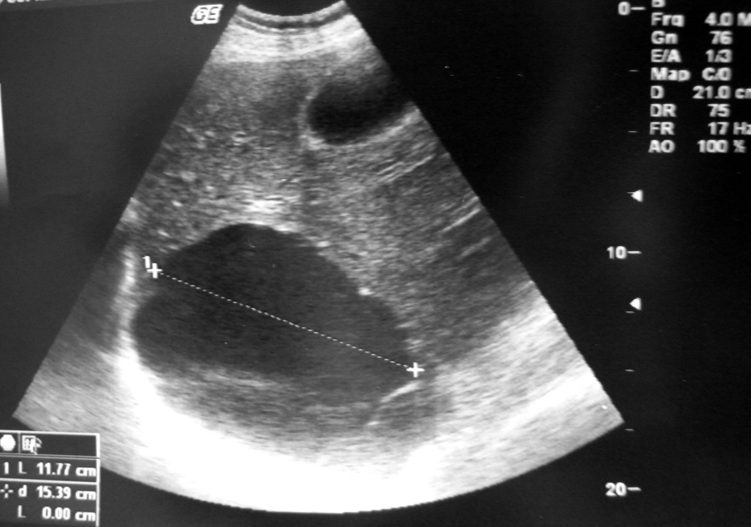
During first admission. Abdominal ultrasound revealing a sizeable right liver lobe cystic mass.
